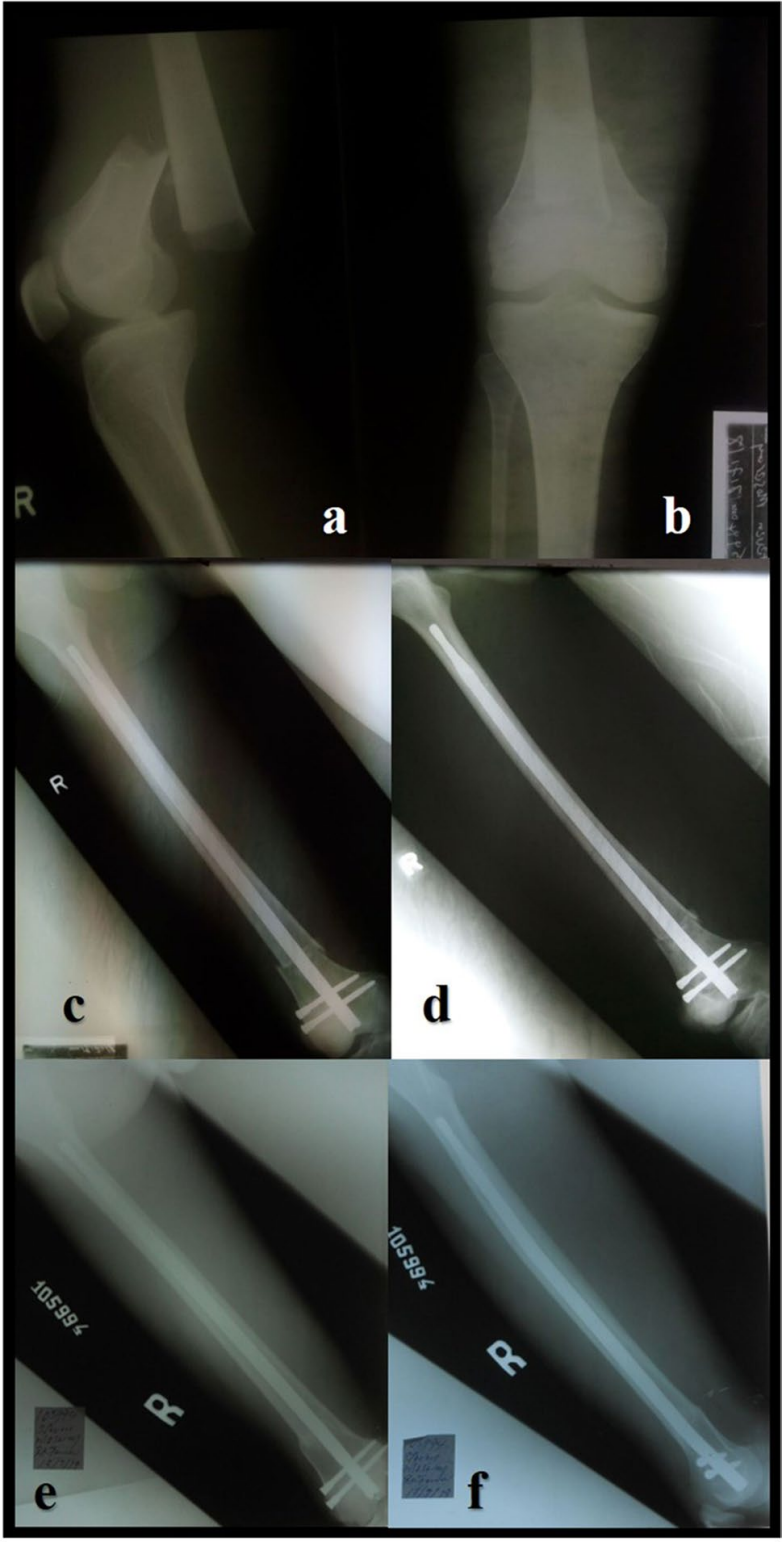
A 18 year old male patient with distal shaft fractures treated using SFN®, (a, b) preoperative radiographs, (c, d) postoperative radiographs, (e, f) consolidation at the follow up at 12 months
